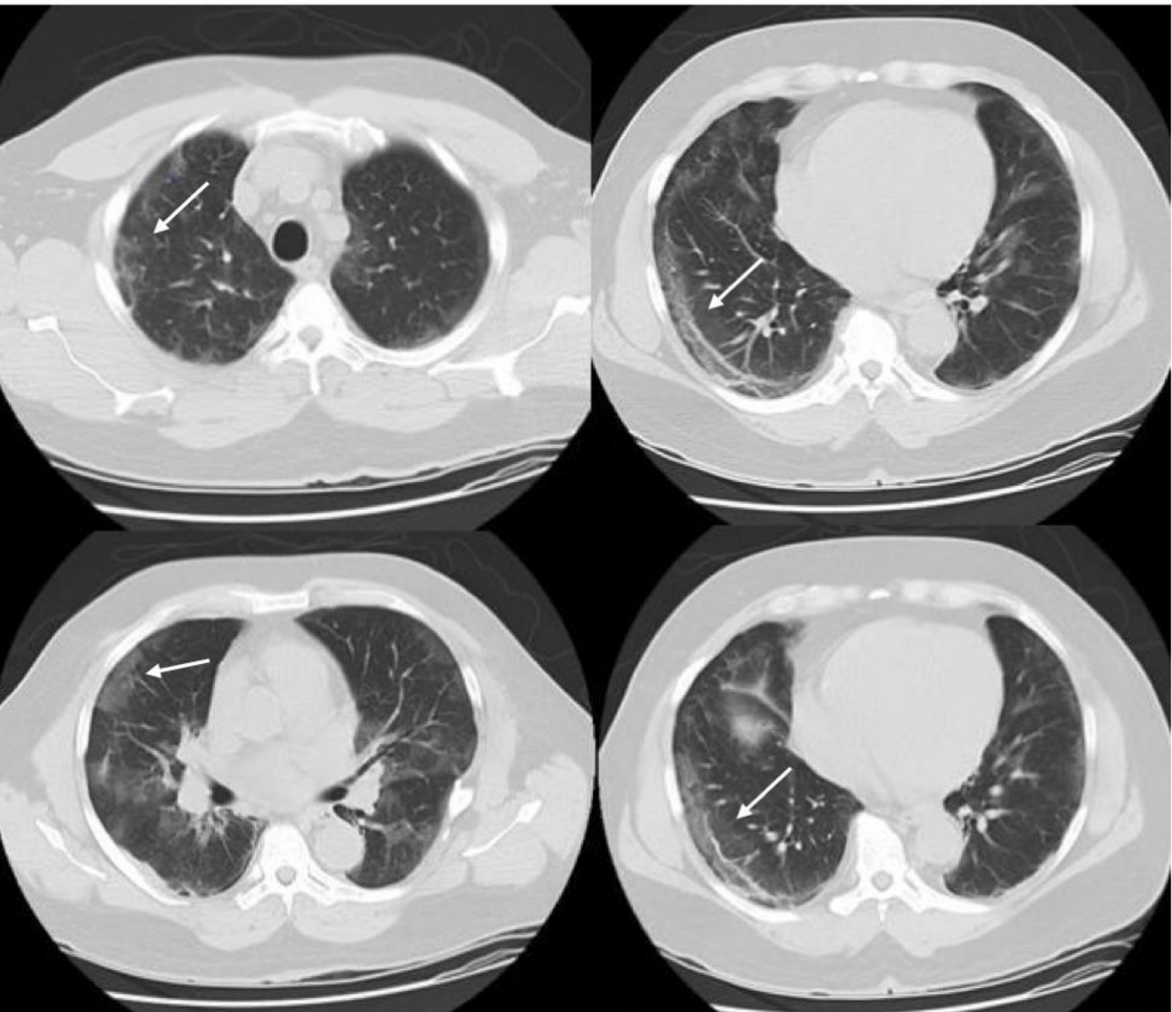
Non-contrast CT scan of the chest Day 1 of hospital admission. Non-contrast CT image of the chest showing multiple, bilateral ground-glass opacities (white arrows) in both upper and lower lobes with a peripheral distribution.
